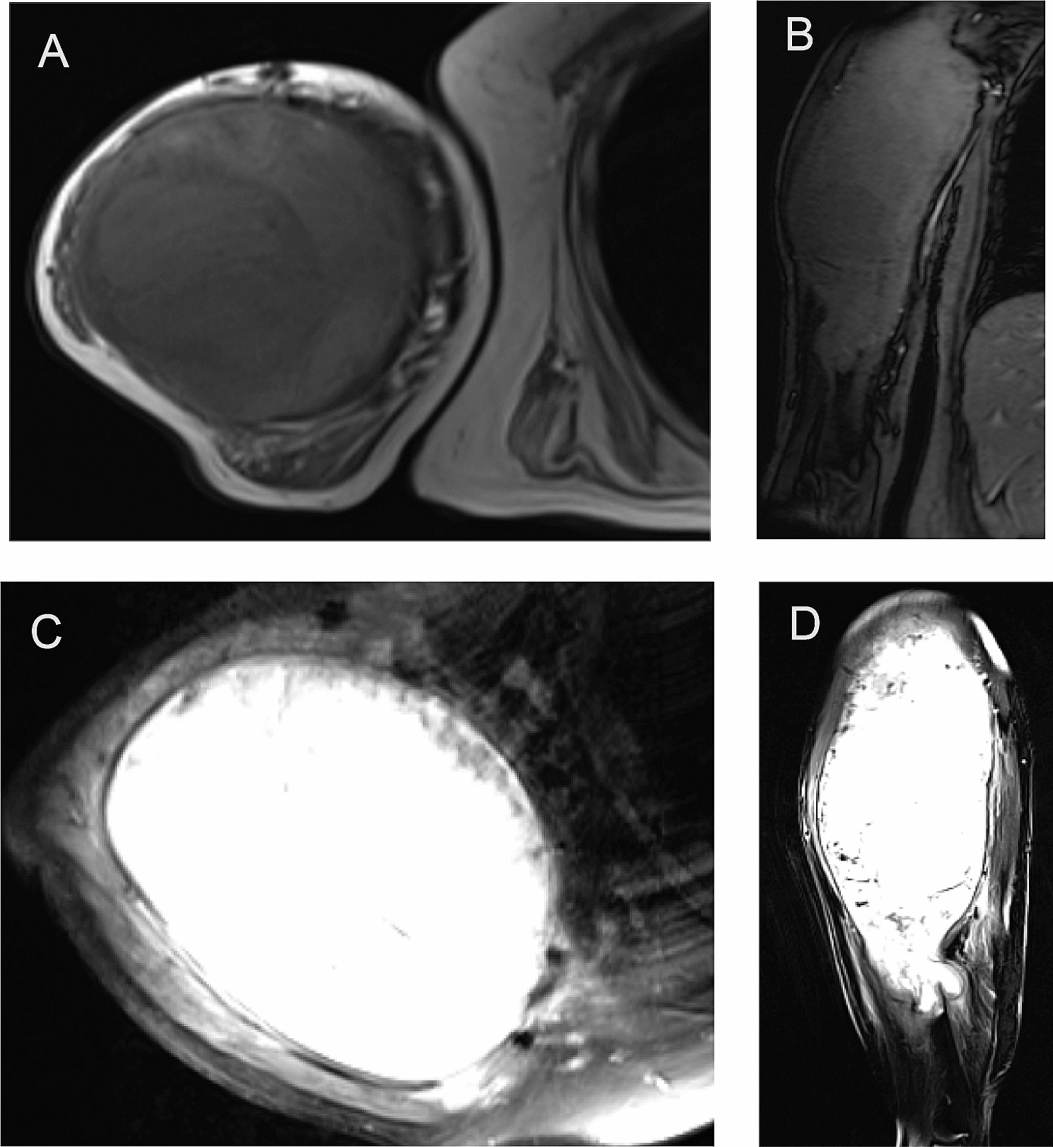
(A, B, C, D) Magnetic resonance imaging (MRI) of the right shoulder and the right upper arm
